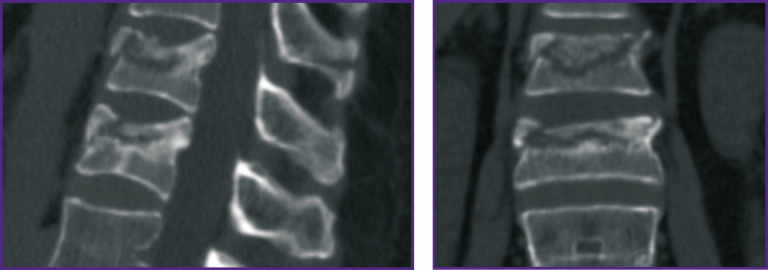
CT scan of Th_12_ and L_1_ vertebrae of patient I. before surgery
